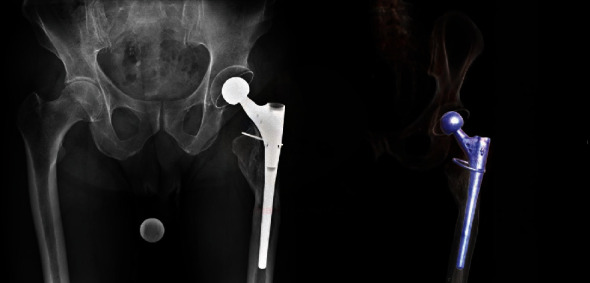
Preoperative AP pelvis X-ray and 3D CT scan showing a well-fixed Wagner-type shaft with excessive bone loss at the area of the greater trochanter (Gruen zones 1 and 2) and diffuse bone remodeling around the medial femoral cortex.
